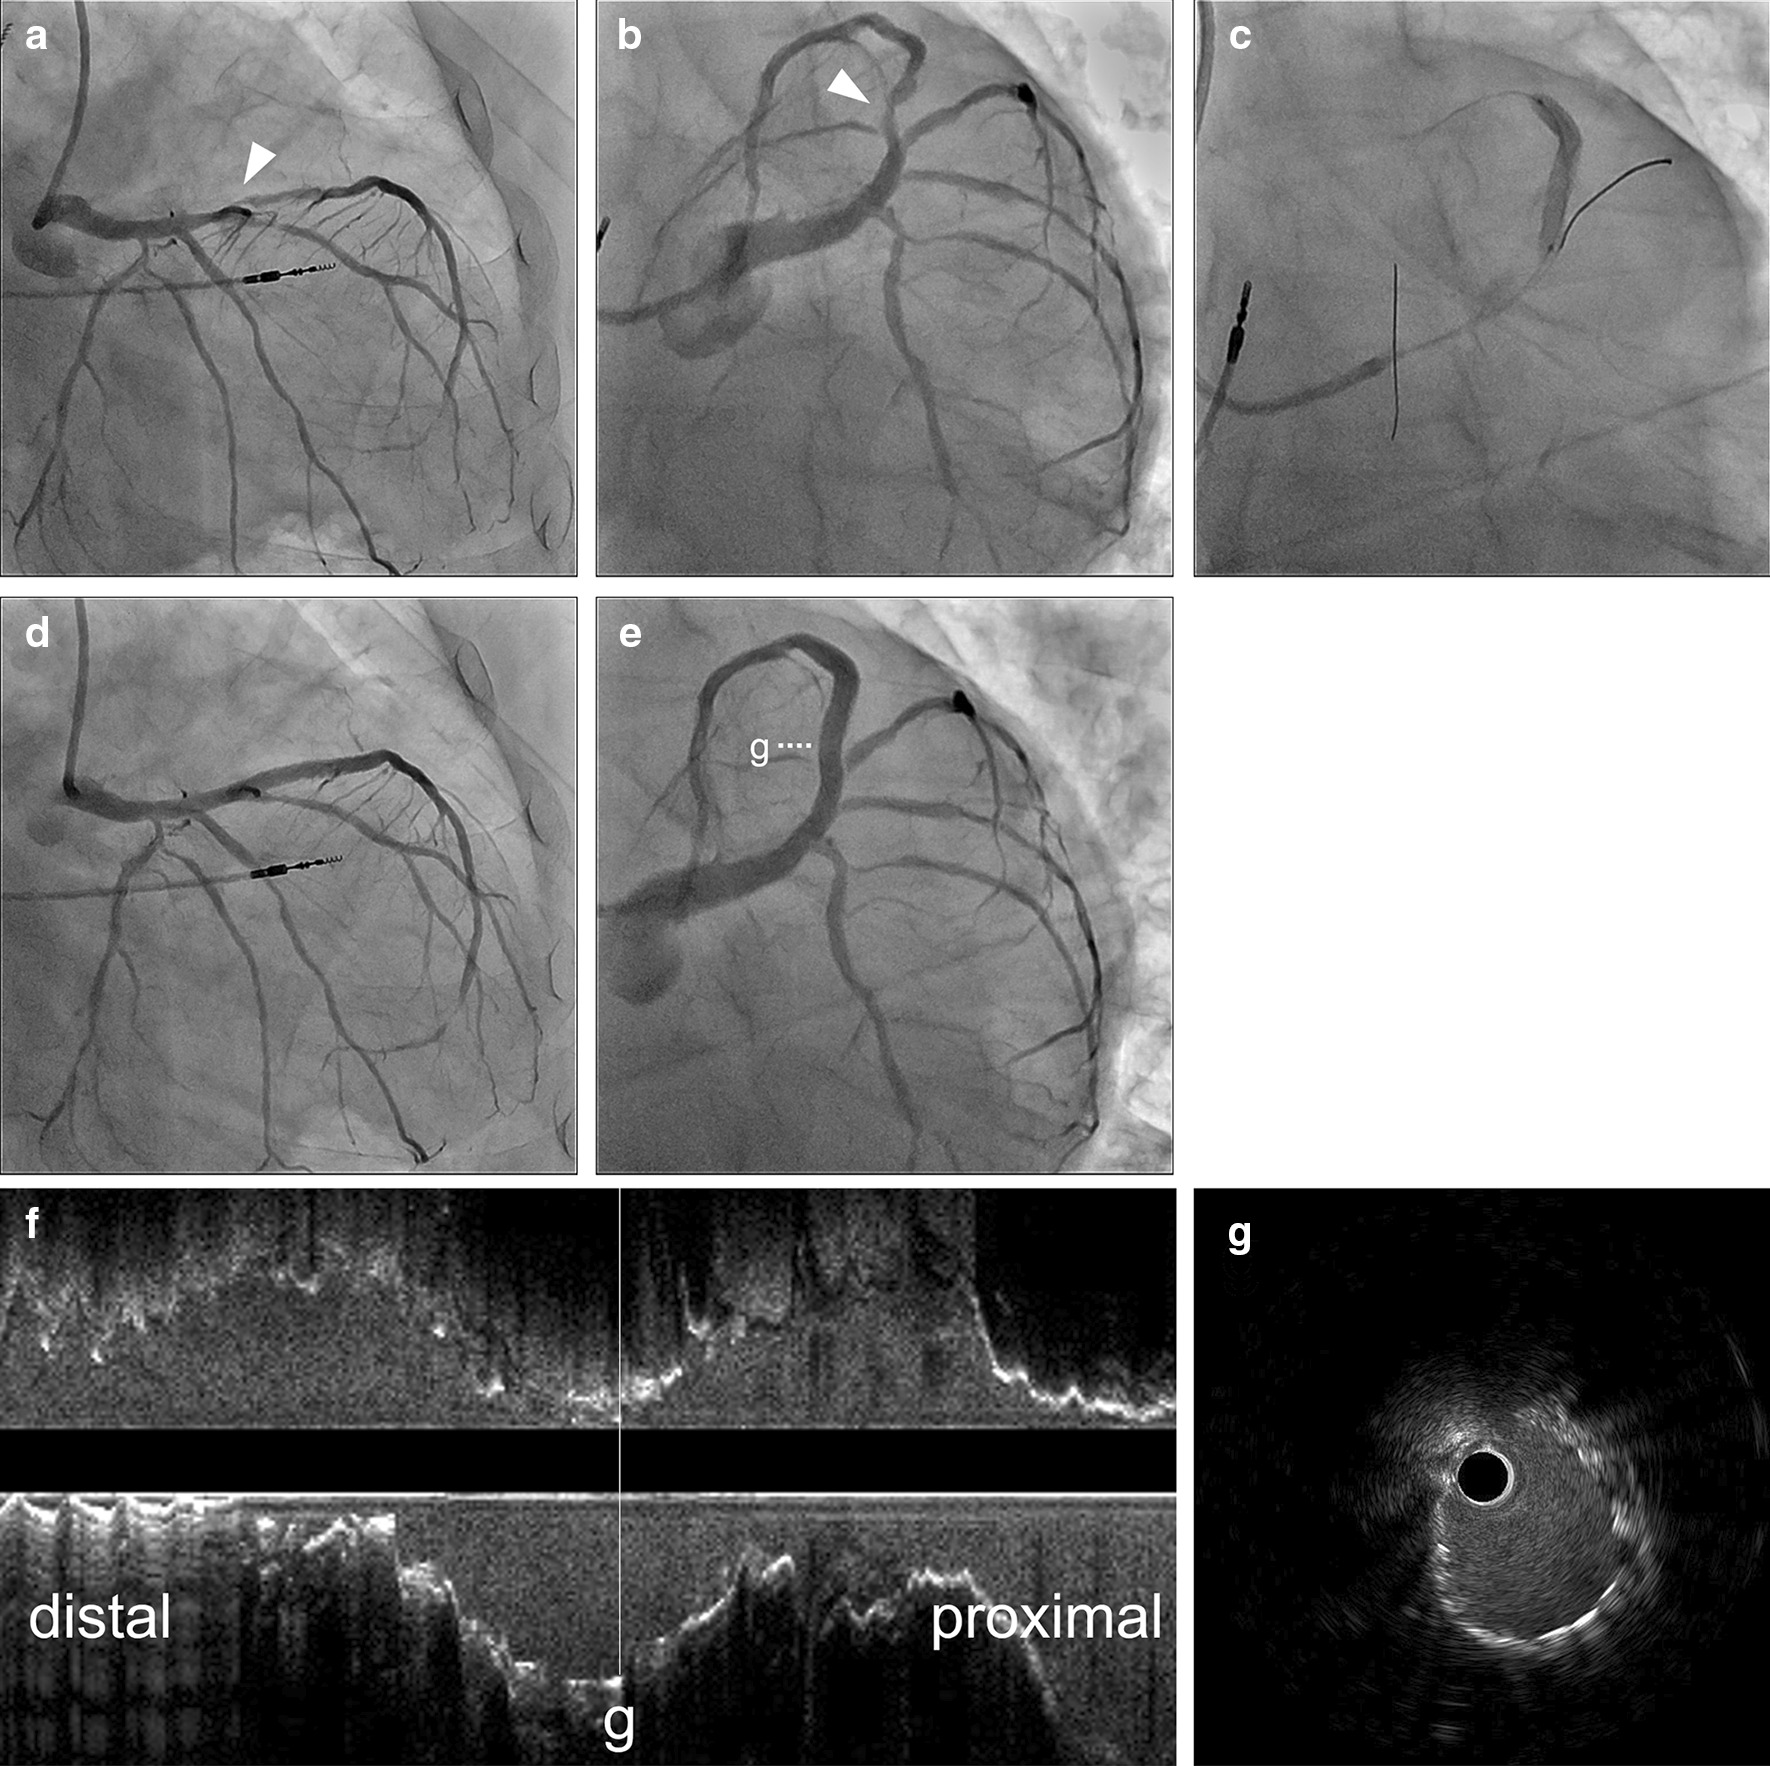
Percutaneous coronary intervention for left anterior descending artery under intravascular ultrasound guidance. a, b Severe stenosis in the left anterior descending (LAD) artery (arrowhead) on initial coronary angiography (CAG). c Stent implantation in the LAD. d–g Final CAG showing an optimal result, with longitudinal and cross-sectional views in intravascular ultrasound imaging; acceptable stent expansion is confirmed
